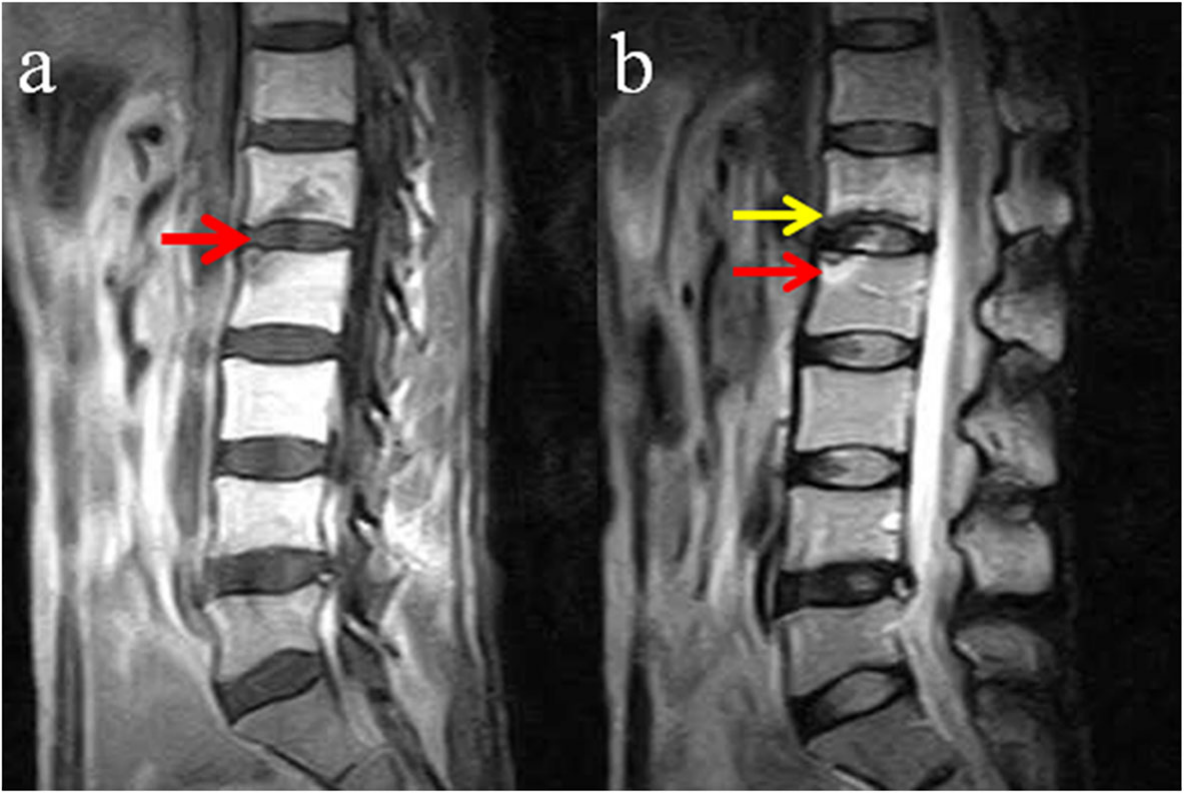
MRI images at 2 years after radiotherapy: a T1WI demonstrating a small hypointense area at the end plates of L1 and L2 (red arrow). b T2WI showing a hypointense signal change at the inferior end plate of L1 (yellow arrow) and high-low mixed signal change at the superior end plate of L2 (red arrow), without obvious signal changes of the intervertebral disk
